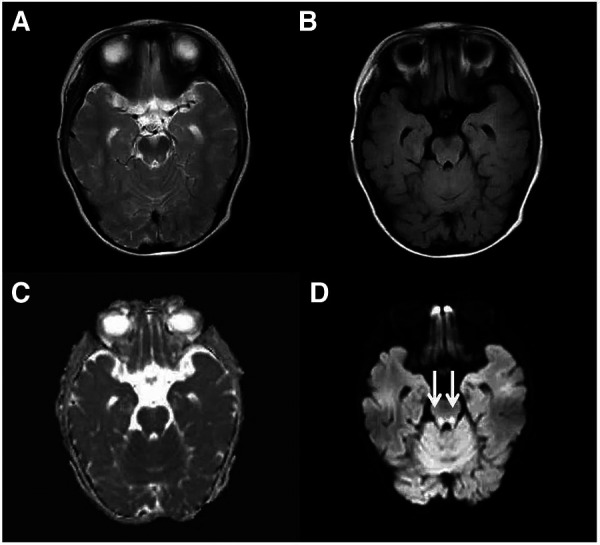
Cranial MRI at the level of the midbrain. An axial T2-weighted (A), an axial FLAIR (B), a dADC (C) and a DWI (D) scans are shown and abnormally high signals in DWI in the midbrain can be seen (white arrow).
